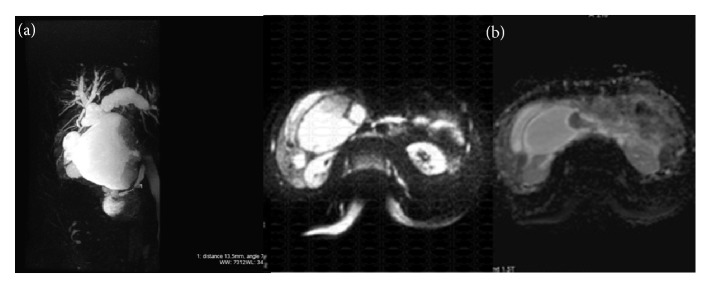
Biliary MRI. (a) Front MIP reconstruction of the bile ducts and cystic mass. (b) The diffusion sequence showed high signal intensity of intracystic bud and the apparent diffusion coefficient (ADC) map shows low signal intensity of lesions characteristic of tumor lesion.
